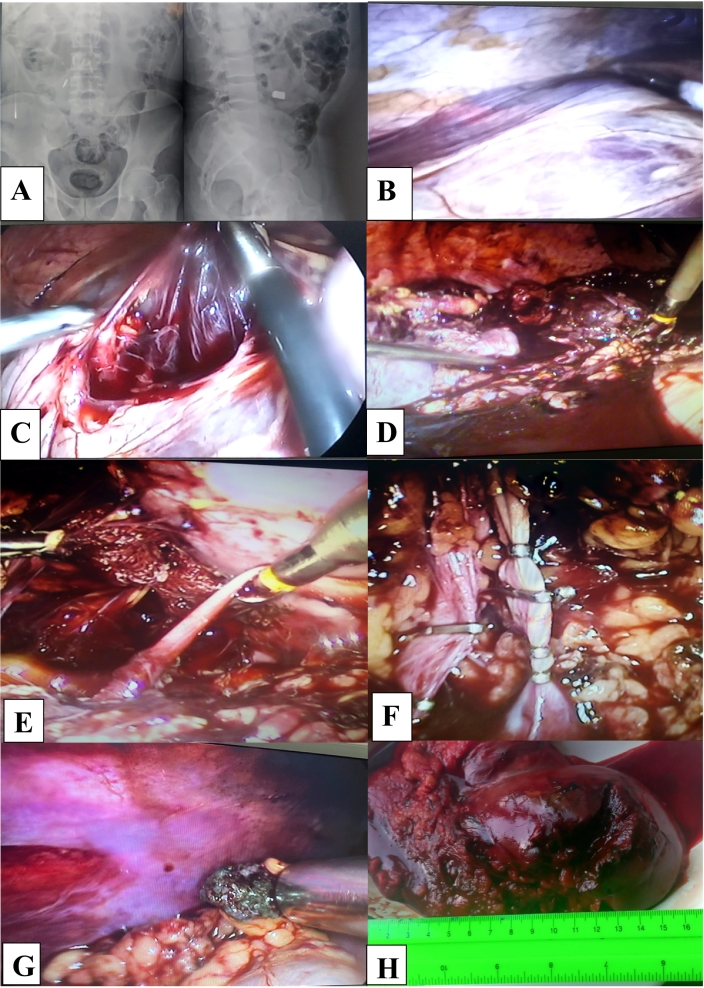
Wounded V., (A - X-ray in the direct and lateral projection of abdominal organs - presence of a foreign body - a metal fragment in the right side of the abdomen; B - Inspection laparoscopy – retroperitoneal hematoma on the right; C - Opening and removal of the retroperitoneal hematoma on the right; D - Bleeding from the right kidney with a firearm shrapnel damage; E - Visualization of the right ureter; F - Clipping of the renal artery and renal vein on the right; G - metal fragment was removed with a magnet instrument during laparoscopy; H - macropreparation, the right kidney was removed due to fire shrapnel damage - V degree according to the AAST classification).
